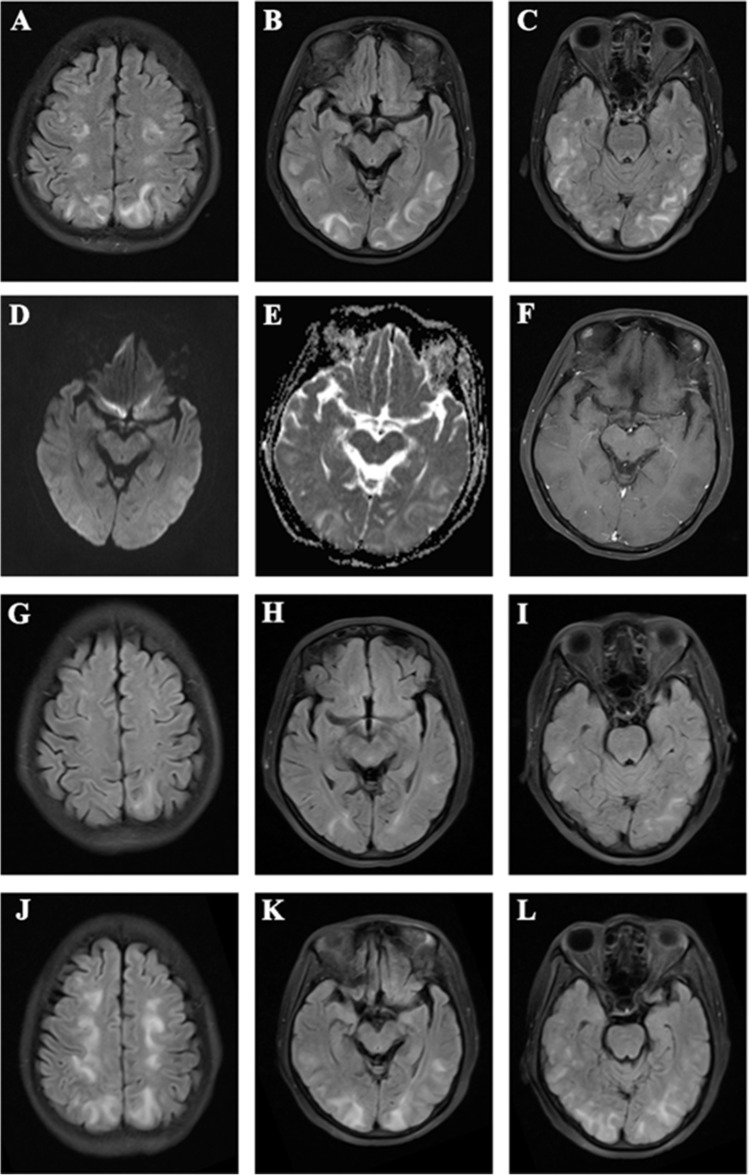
Brain MRI showing findings consistent with posterior reversible encephalopathy syndrome. A–C Increased symmetric signals on T2-Flair-weighted images of the parieto-occipital lobes. D and E Hyperintense lesions on DWI and increased ADC values. F Contrast-enhanced MRI scans show no enhanced signals. G–I. T2-Flair-weighted images after therapy. J–L Cerebral lesions recurred after a 4 mg dose of fruquintinib was restored
